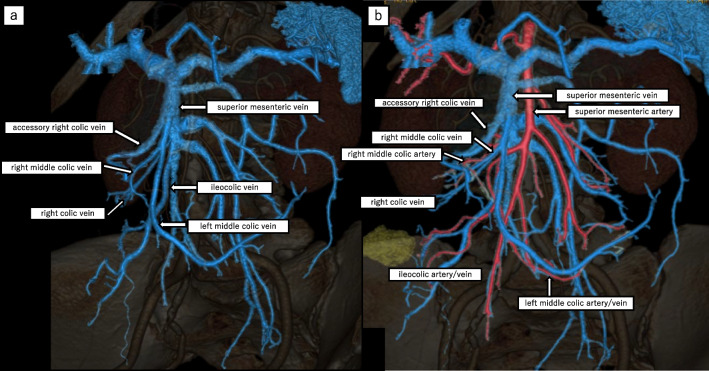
3D-CTA image (a vein, b artery and vein). a 3D-CTA confirmed that the RCV, MCV and ICV arise from SMV. b The relationship between veins and arteries
